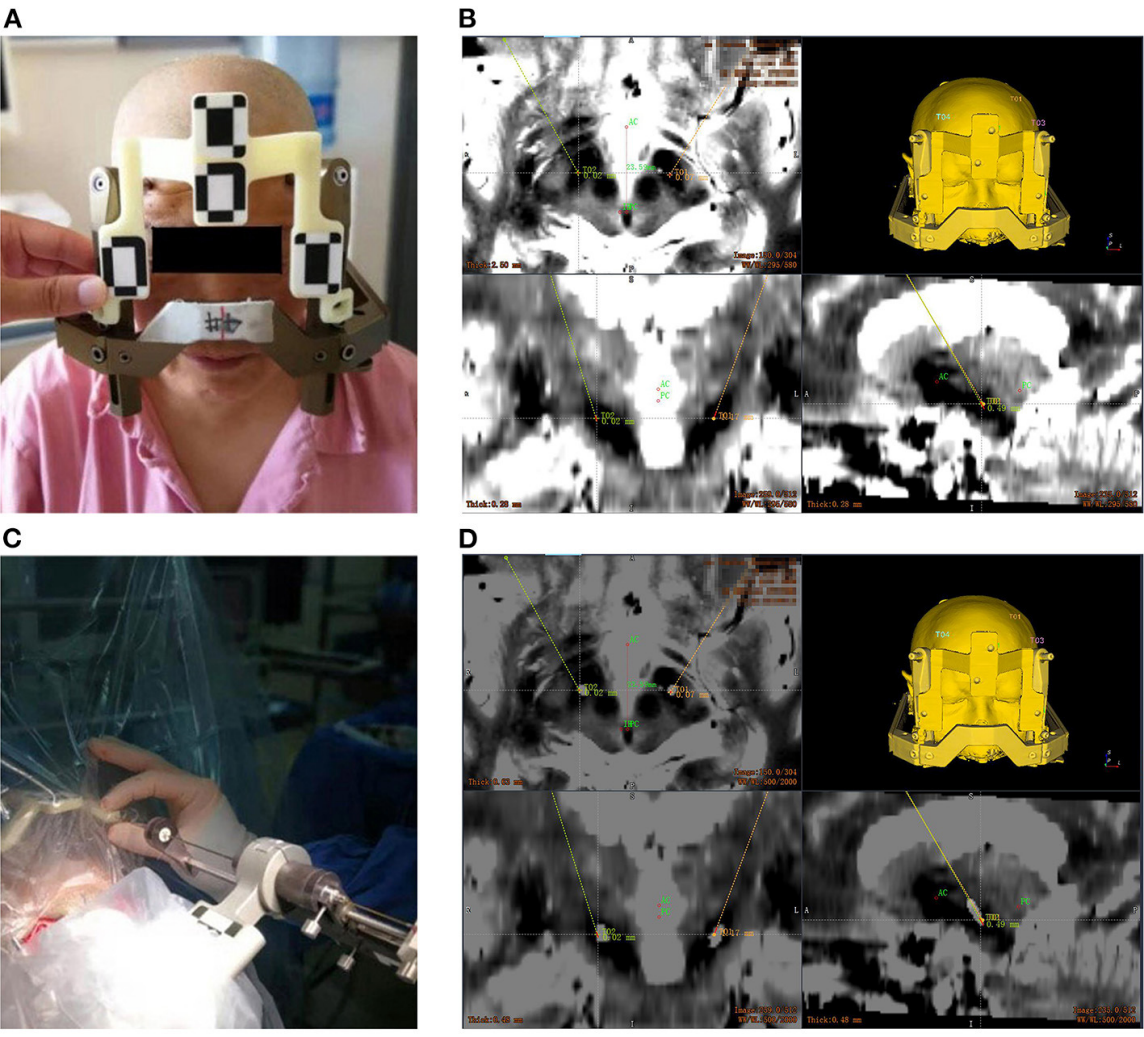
Electrode implantation in a DBS procedure assisted by the Remebot robotic system. (A) The optical marker screwed to the Leksell frame; (B) the target points and trajectories planned upon the fusion of preoperative MR and CT images; (C) the verification of accuracy after the automatic optical registration; (D) overlay of the postoperative CT images and preoperative MR images with bilateral target trajectories.
